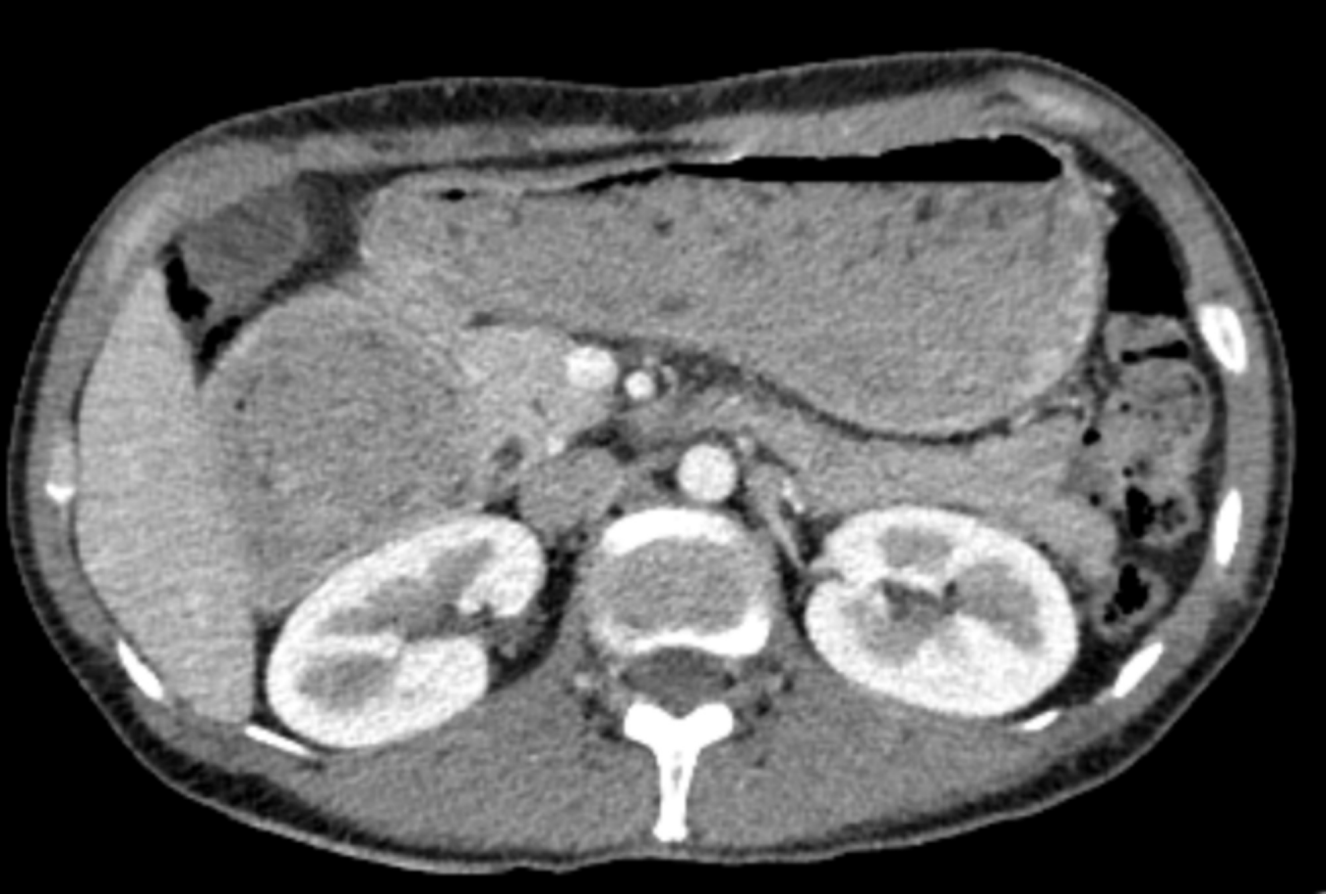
Imaging after completion of treatment Computed tomography image showing resolution of the peritoneal carcinomatosis after 6 cycles of chemotherapy
